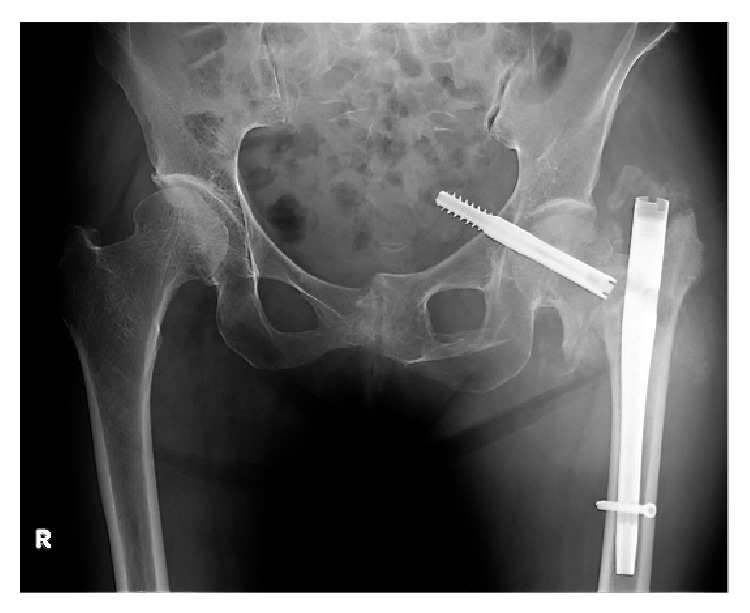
Radiograph showing redisplacement of the fracture and intrapelvic migration of the lag screw through the femoral head and the medial wall of the acetabulum.
